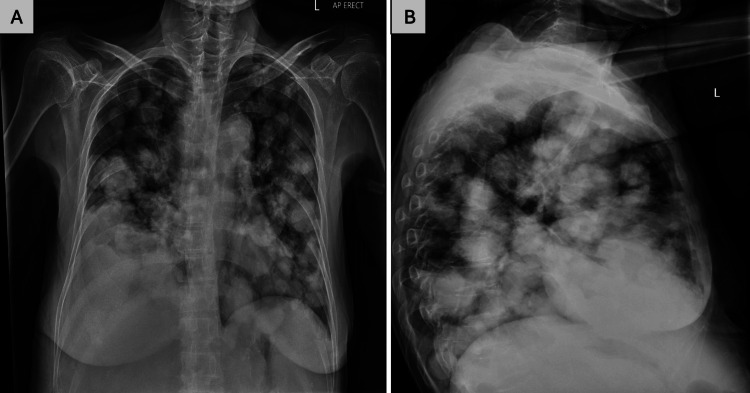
Chest radiography images showing multiple large and round nodules
